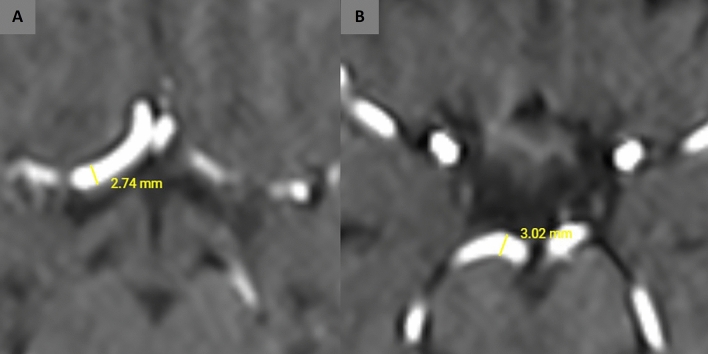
The measurements of vascular diameters of the right A1 segment (A) and right P1 segment (B) were shown. Axial MR images with ×400 magnification were used to measure the vessel calibers.
